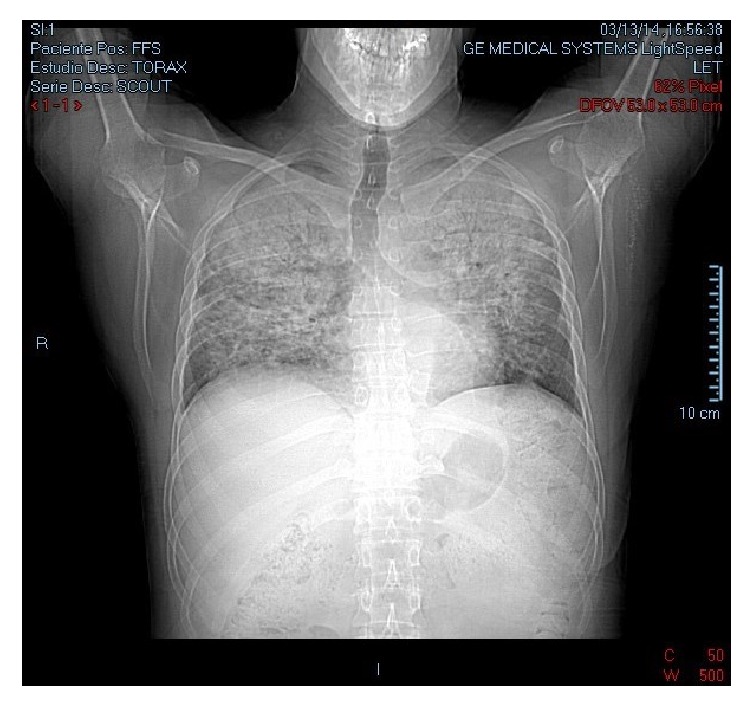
Pulmonary CT-scan with evidence of atypical bilateral pneumonia: pulmonary parenchyma with multiple areas of interstitial infiltrates (patchy infiltrates) involving apical and medial areas of both pulmonary fields.
